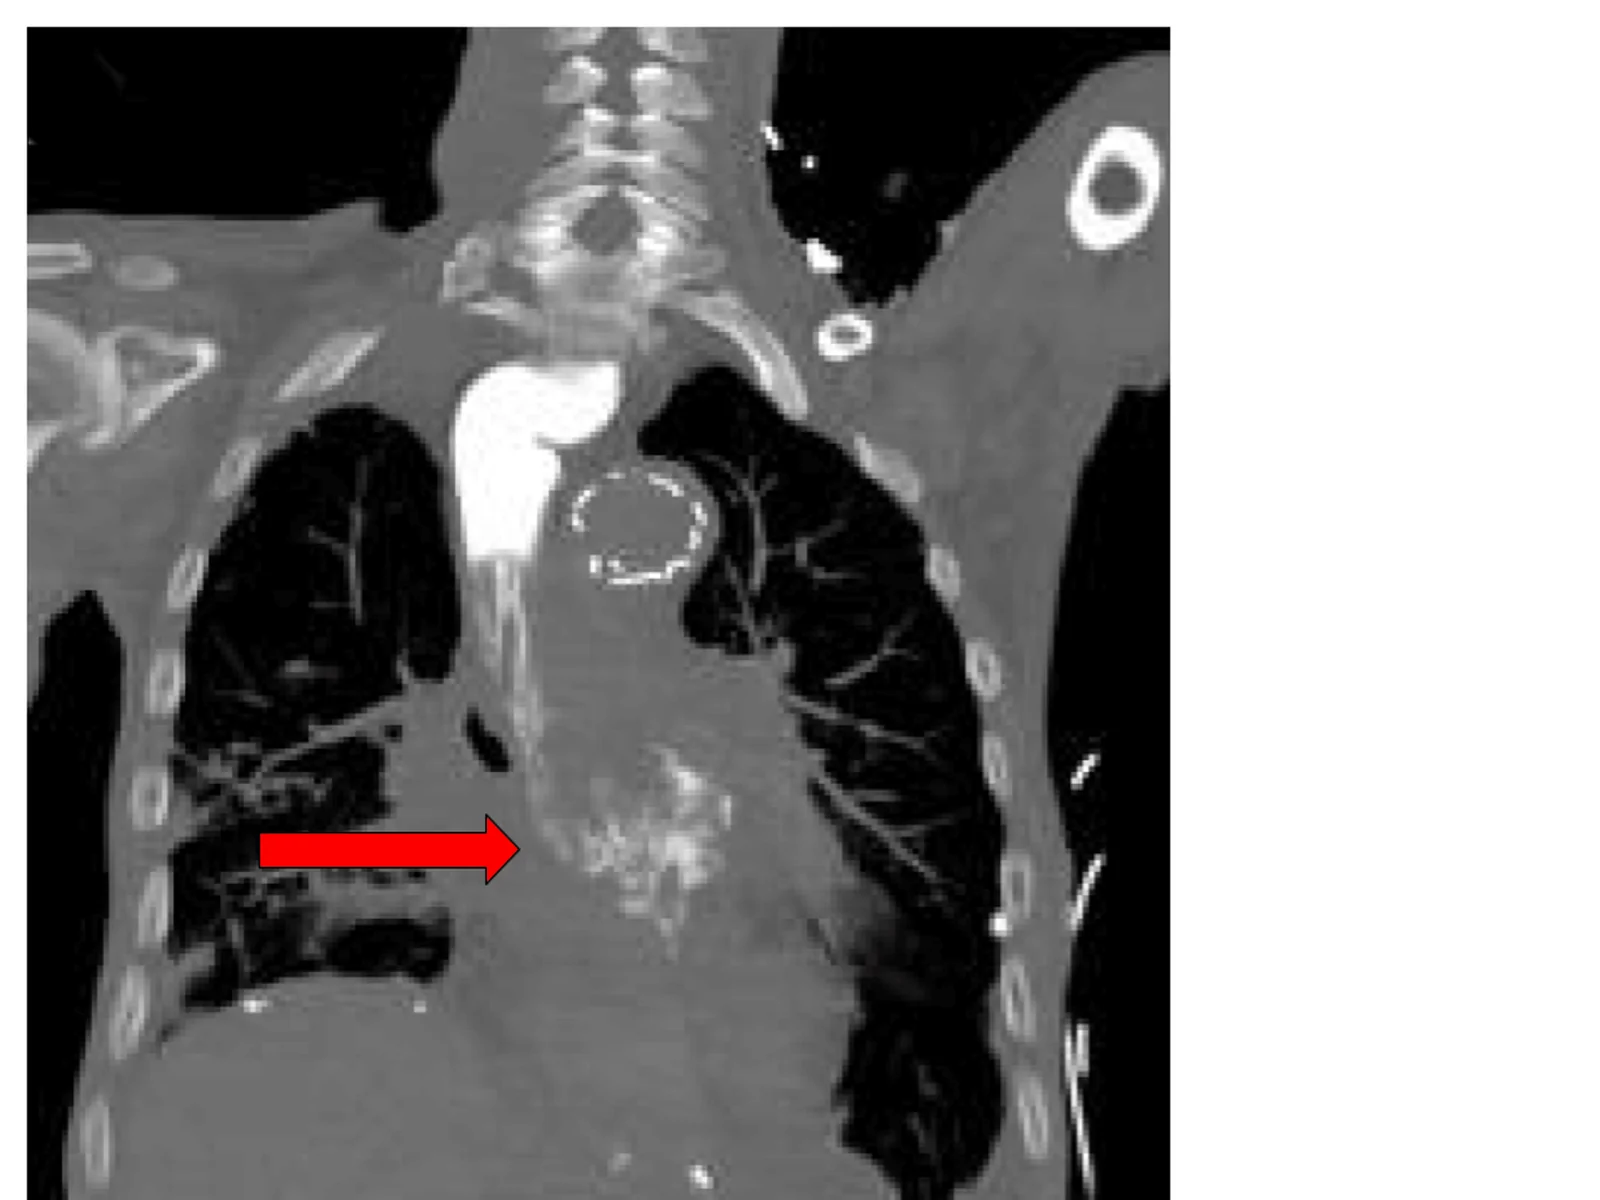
Coronal CT reconstruction after gastrografin administration
